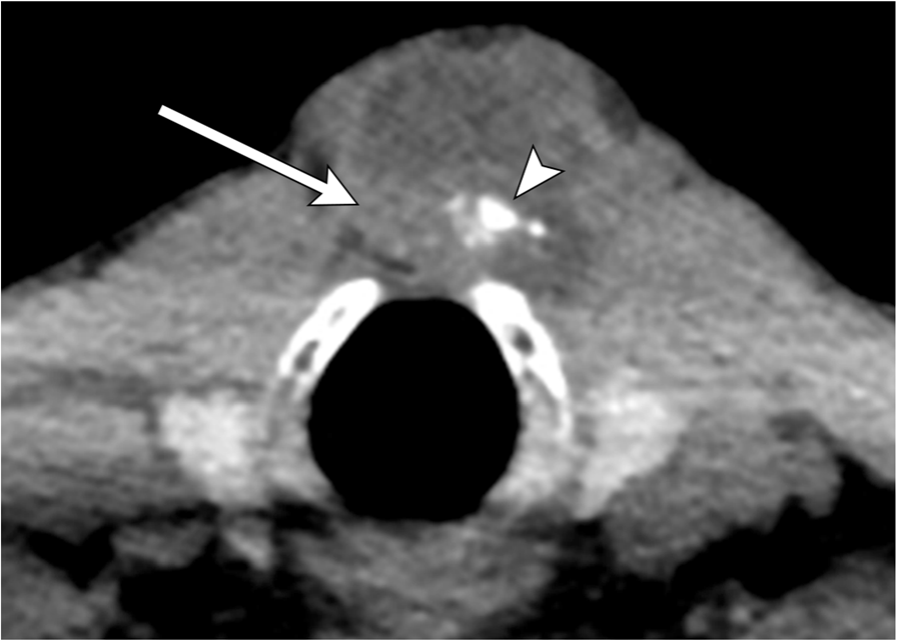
Thyroglossal duct cyst with malignancy. Axial CT image demonstrates a thyroglossal duct cyst, which is predominantly cystic, but also has soft tissue attenuation (arrow) and calcifications (arrowhead) at the posterior aspect. Resection revealed papillary carcinoma
